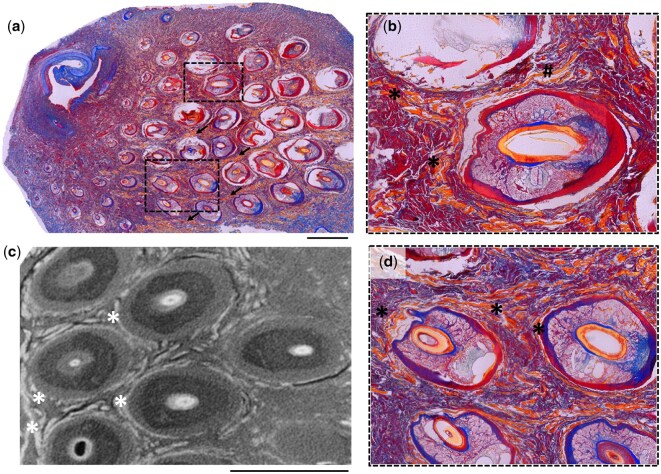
Intrinsic musculature of the mystacial pad of Eurasian Otter (*Lutra lutra*). a) A sagittal cross-section across the pad, showing individual follicles and some intrinsic muscles. Inset b) shows intrinsic muscles in the dorsal, more disorganized area of the pad, where the intrinsic muscles of 2 whiskers from different rows touch. Inset d) shows intrinsic muscles within the more ventral organized area, with intrinsic muscles of 2 neighboring follicles within the same row joining. Panel c shows a micro CT image of the follicles and intrinsic muscles from a dorsal area of the pad, also showing the touching of the intrinsic muscles between rows. Asterisks (*) indicate the intrinsic muscles; hashes (#) the irregular intrinsic muscles; and arrows point to the extrinsic Pars maxillaris muscles. Scale bars are 10 mm.
